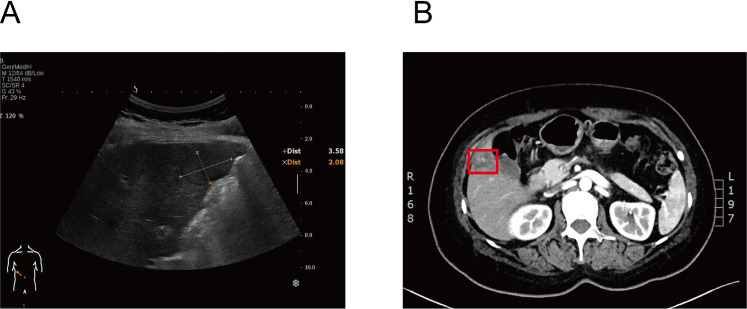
Metastatic imaging. (A) Ultrasound (May 2, 2021): The liver was grossly normal in size and contour. A well-circumscribed solid hypoechoic nodule (~3.5 × 2.1 cm) was identified near the gallbladder fossa with heterogeneous internal echotexture. (B) Contrast-enhanced CT (May 2, 2021): CT demonstrated a hypodense lesion in hepatic segment V (~3.0 × 2.7 cm, inside the red box) with prominent rim enhancement and central necrosis, associated with focal capsular bulging.
